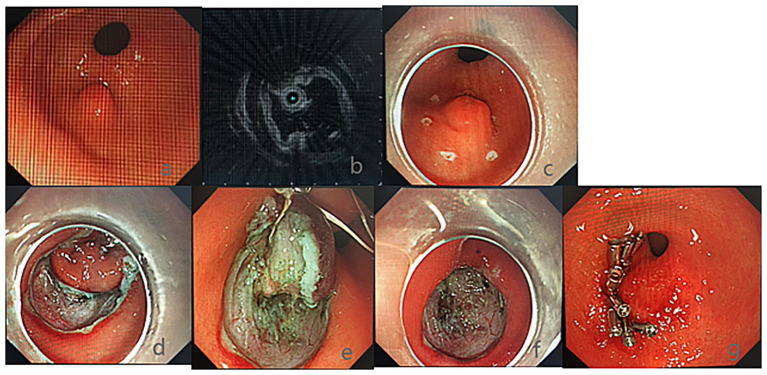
Endoscopic and ultrasonographic workflow for diagnosis and treatment of upper gastrointestinal submucosal lesions. Image brightness and contrast were uniformly adjusted using standard medical image editing software (e.g., Adobe Photoshop) to enhance clarity for publication; these adjustments did not alter the diagnostic features of the lesions. (a) Under white light endoscopy, a 0.8 cm submucosal protrusion is seen in the antrum of the stomach with a smooth mucosal surface, no ulceration, or hyperemia. (b) Endoscopic ultrasonography shows the lesion originating from the submucosal layer, with a hypoechoic, homogeneous texture and a visible tubular opening (arrow), suggestive of ectopic pancreas. (c) Marking the lesion boundary using a dual knife (Olympus KD-650L) at a 5-mm distance from the lesion edge to ensure complete resection. (d–f) Complete stripping of the lesion along the edge of the lesion with stepwise submucosal dissection; continuous hemostasis is performed using hot biopsy forceps (Olympus FB-25K-1) to prevent bleeding. (g) Metal clips (Olympus HX-610-135 L) closing the 1.0-cm mucosal defect to avoid perforation or delayed bleeding.
